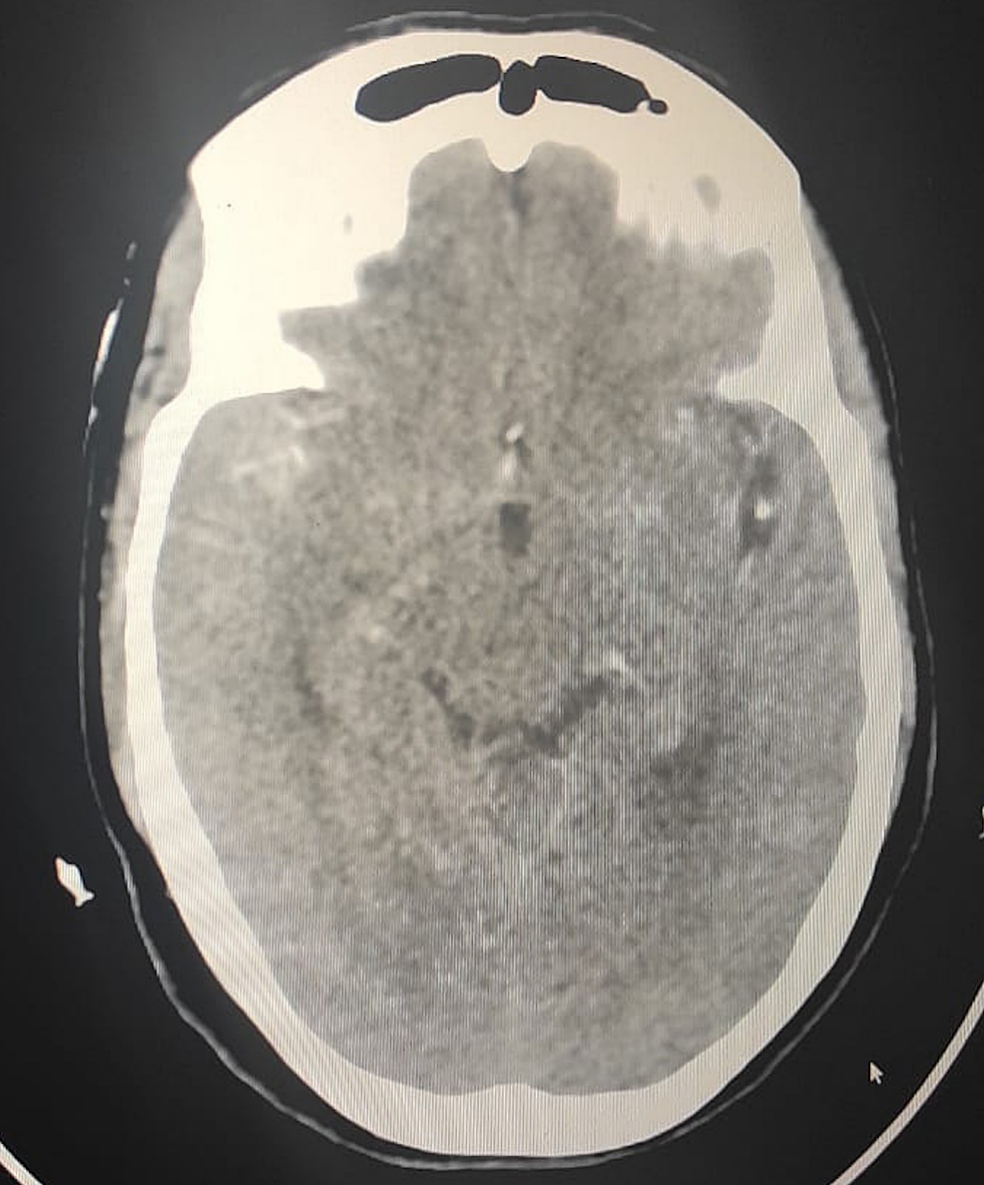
Plain CT scan of the brain showing a subarachnoid hemorrhage CT, Computed tomography.
